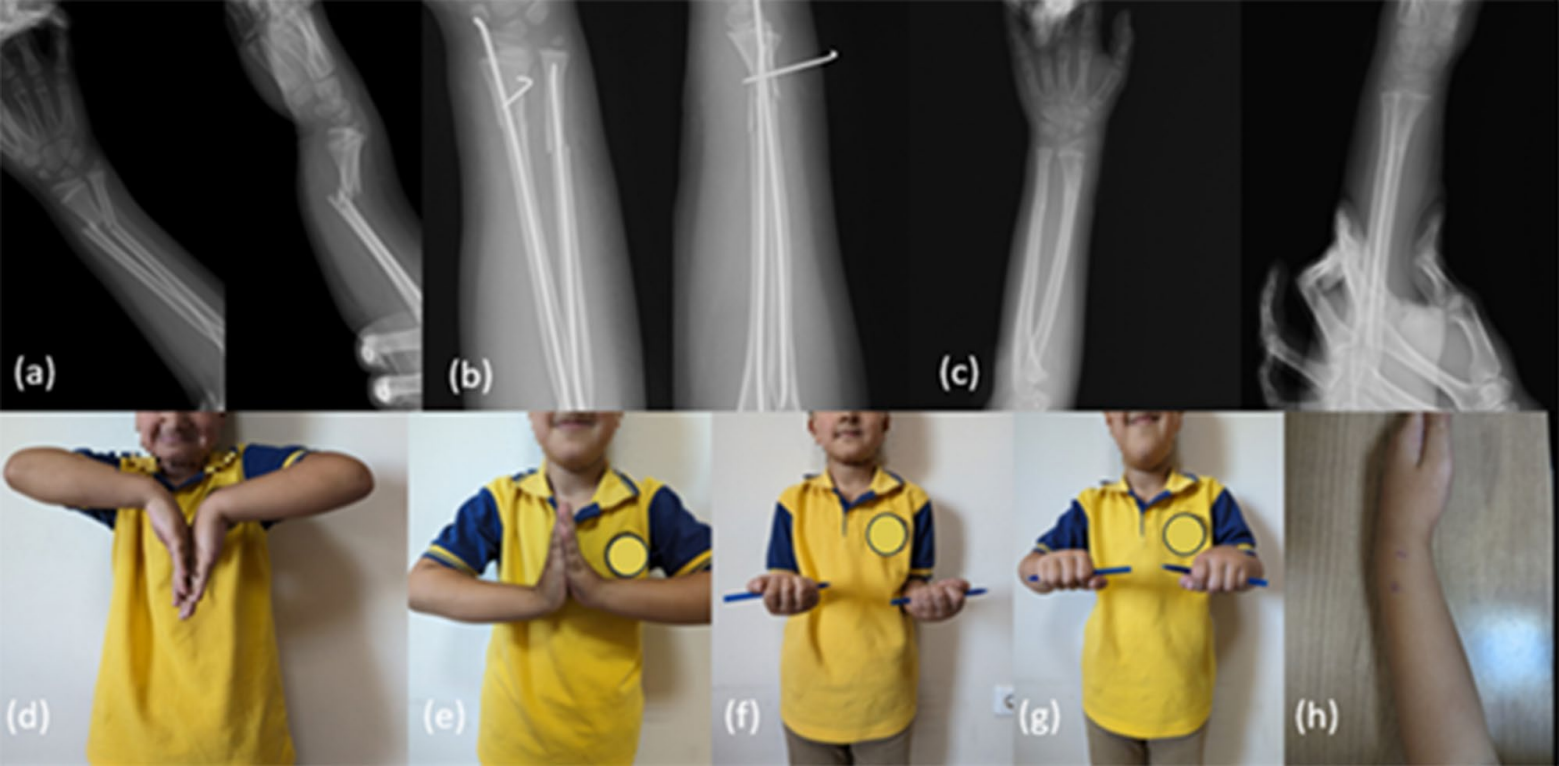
A male patient, 11 years old, with a left DRDMJ fracture accompanied by an ulna fracture. Anterior-posterior and lateral radiographs: (a) preoperative; (b) day one postoperative; (c) 3 months postoperative. Wrist and forearm range of motion examination at last follow-up: (d) flexion; (e) extension; (f) supination; (g) pronation; (h) scar
